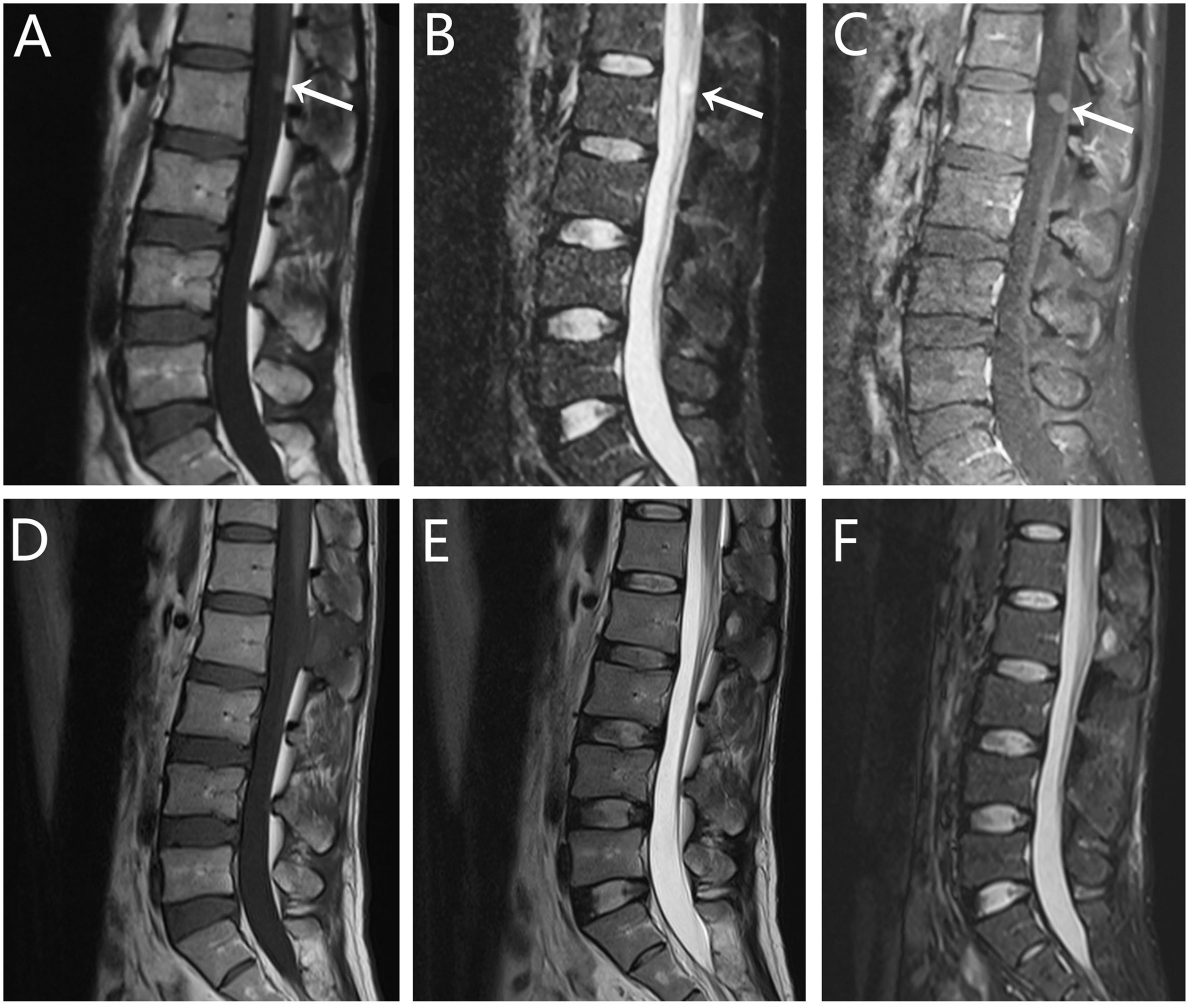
Radiographic images of case 1. (A–C) Pre- and (D–F) postoperative magnetic resonance imaging (MRI) scans revealed a cystic lesion dorsal to the L2 spinal cord. Sagittal MRI scan demonstrated isointense signal on (A) T1-weighted imaging (T1WI) and hyperintense signal on (B) T2-weighted imaging (T2WI). (C) T1WI with gadolinium administration showed slightly enhanced. After 11 months of follow-up, the re-examination MRI showed that the lesion was totally resected, with no evidence of recurrence.
